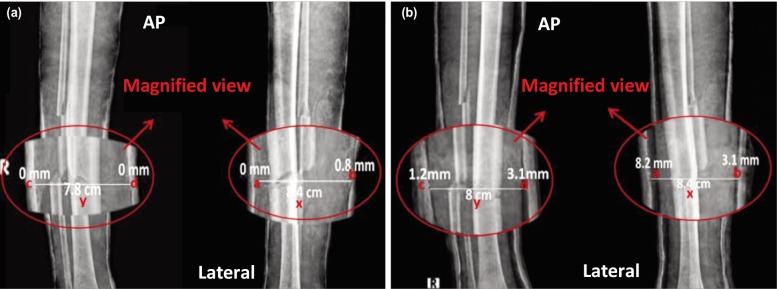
Radiographs of fracture site (a) Immediate post-reduction and (b) At 3 weeks follow-up.
